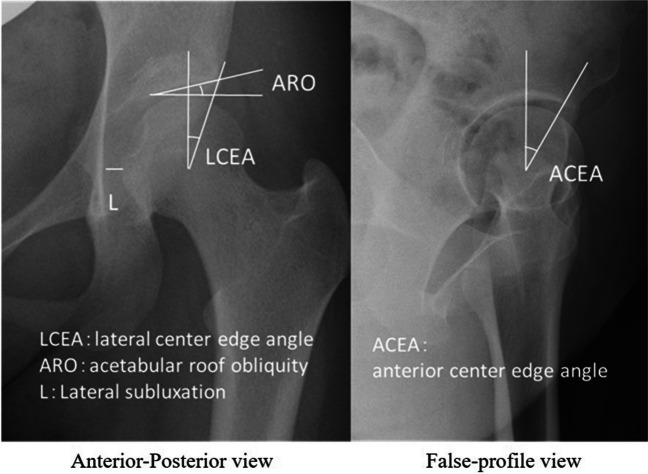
Radiographic evaluations
